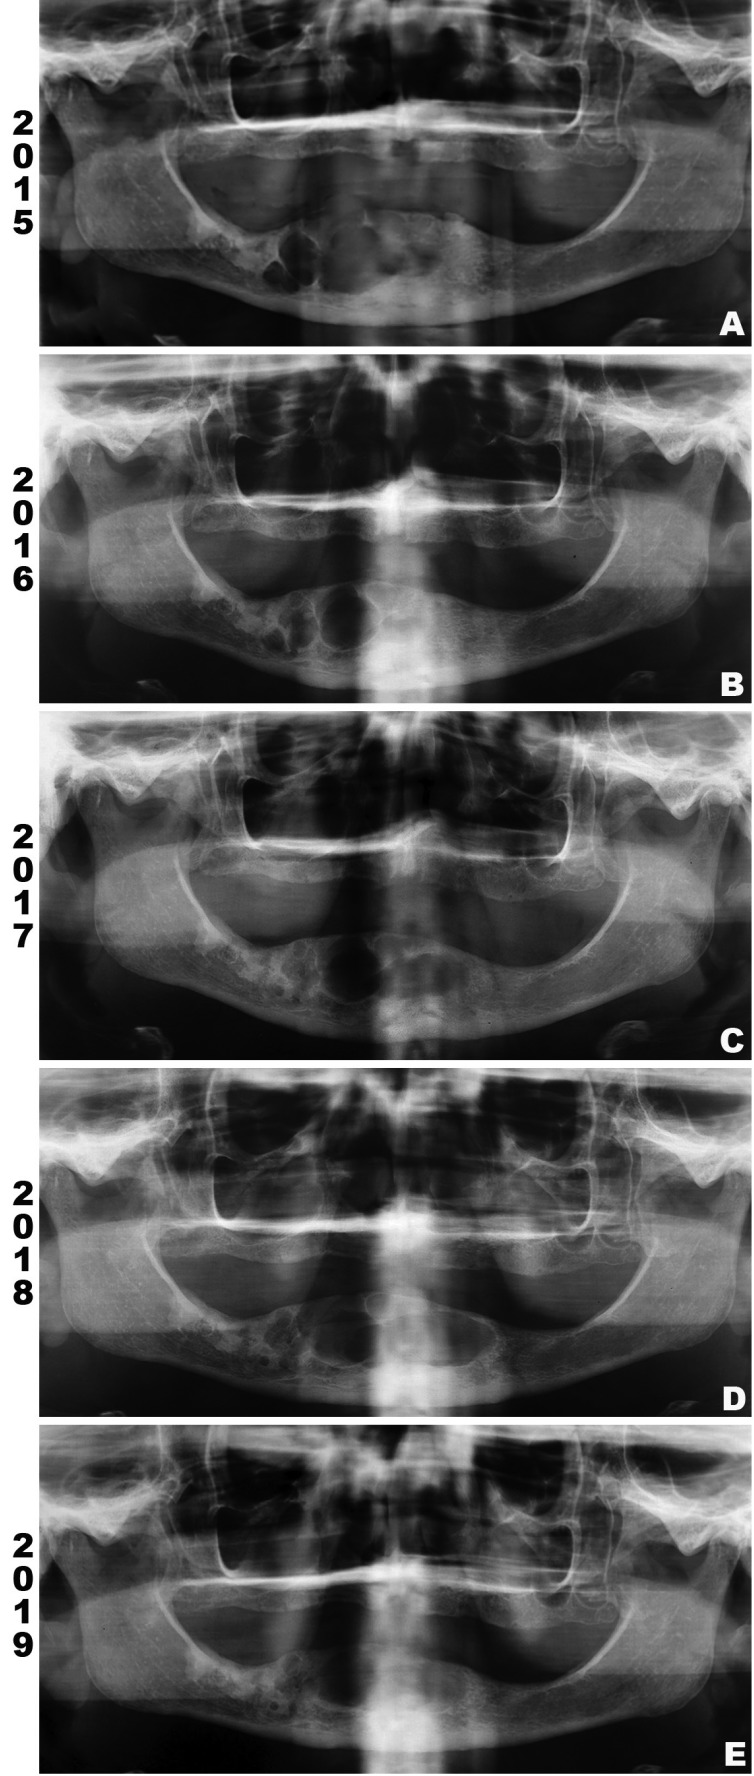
Panoramic radiograph (PR). (A) PR taken in 2015 showing a large, multilocular, well-defined radiolucent lesion extending from the region of left mandibular lateral incisor to right mandibular first molar teeth. (B) PR taken in 2016 indicating decreases of the lesion on right posterior region of mandible. (C and D) PR from 2017 and 2018, respectively, revealing increase of osteolytic component on anterior region of mandible, extending to the left side. (E) PR from 2019 shows increased radiopacity of the lesion, indicating bone repair.
